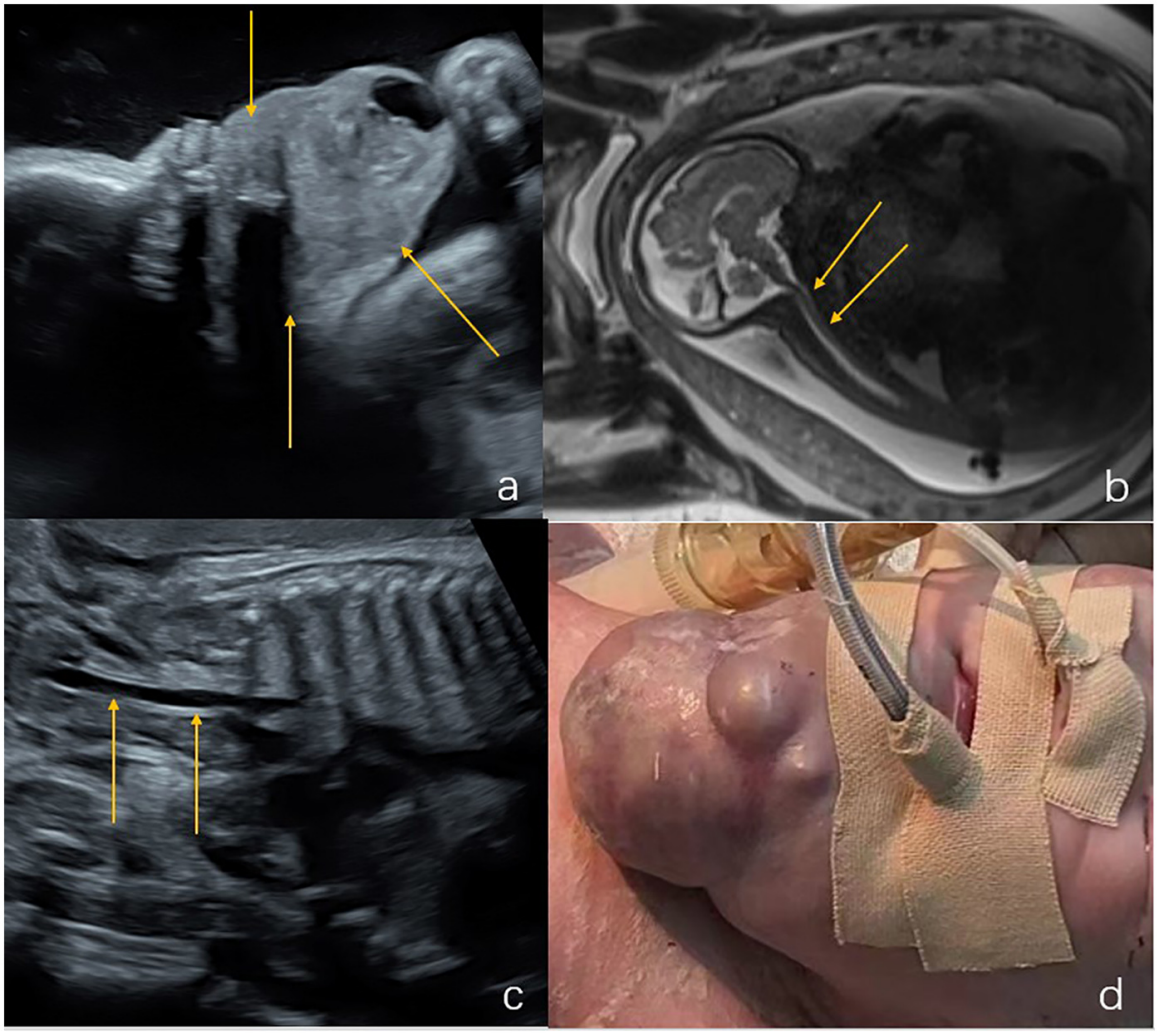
Hemangioma in the neck of a fetus (case 16). (a) Ultrasound examination at 28 weeks of gestation revealed a solid mass (6.9 cm × 5.5 cm × 5 cm) in the submandibular region and neck (indicated by the arrows). (b) Sagittal T2-weighted magnetic resonance imaging, the structure of the entire trachea was visible (indicated by the arrows). (c) Ultrasound examination at 37 weeks of gestation showed that the mass was 7.2 cm × 6.0 cm × 5.9 cm. The trachea was visible (indicated by the arrow), and an elective cesarean section was performed at 37 weeks + 3 days of gestation. (d) The tracheal intubation was successful in ex utero intrapartum treatment during delivery. The neck mass of the newborn is shown by the arrow. The mass was surgically removed 30 days after birth and was pathologically diagnosed as a hemangioma with a favorable outcome.
